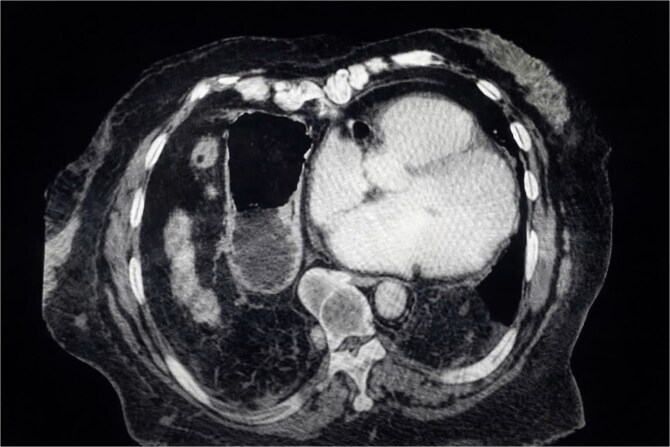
CT scan showing a large right anterolateral diaphragmatic hernia with a maximal *craniocaudal extent*.
